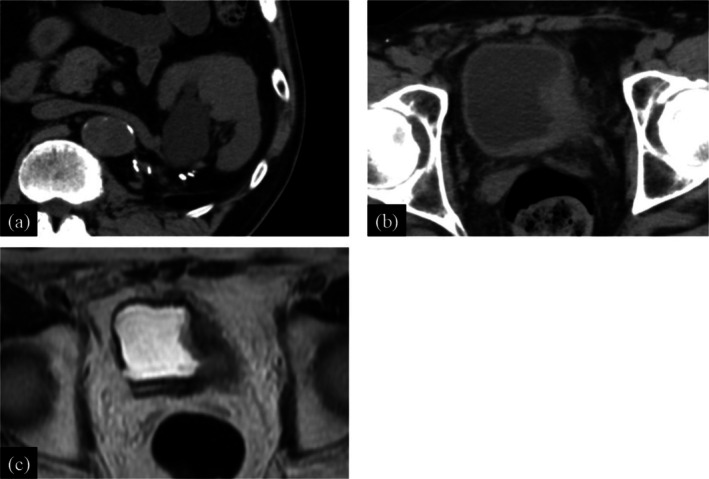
Plain CT images (a, b) and plain MRI image (c) 4 months after the start of anti‐tuberculosis therapy. (a) The size of the left kidney tumor has decreased, but hydronephrosis has appeared. (b) The size of the tumor on the left lateral wall of the bladder has increased. (c) The tumor on the left wall of the bladder shows extramural invasion.
